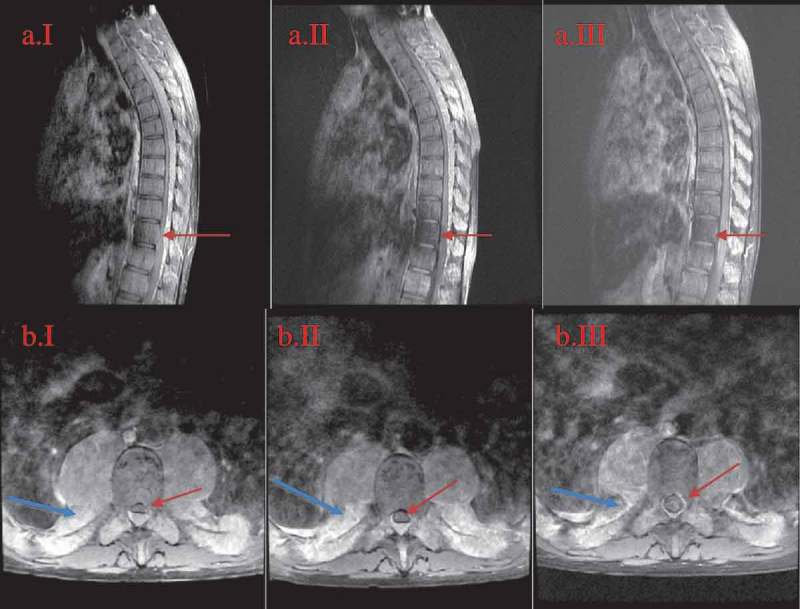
MRI of the thoracic spine in sagittal and axial views at the levels of T6-T7. A = sagittal view; B = axial view; I = initial presentation; II = day 14th after diagnosis; III = week 11th after diagnosis. The regression of the canal stenosis over time from initial presentation to following up can be appreciated on the sagittal view (red arrow) as well as on axial images (red arrow). The reduction of the lesion size can be appreciated on the axial images (blue arrow).
